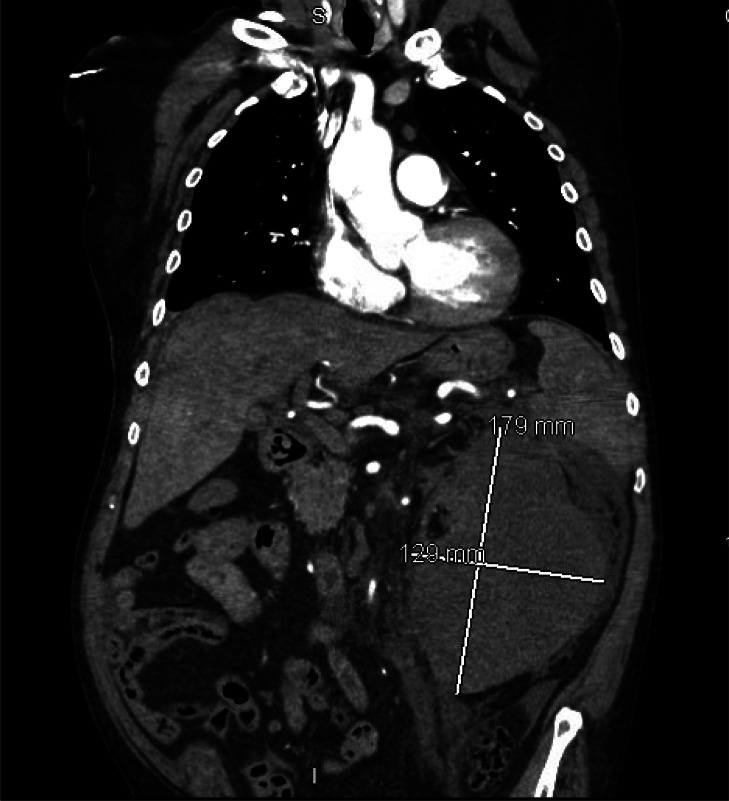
Coronal view of computed tomography angiogram of the abdomen and pelvis showing large left renal subcapsular hematoma and hemorrhage. *mm*, millimeter.
